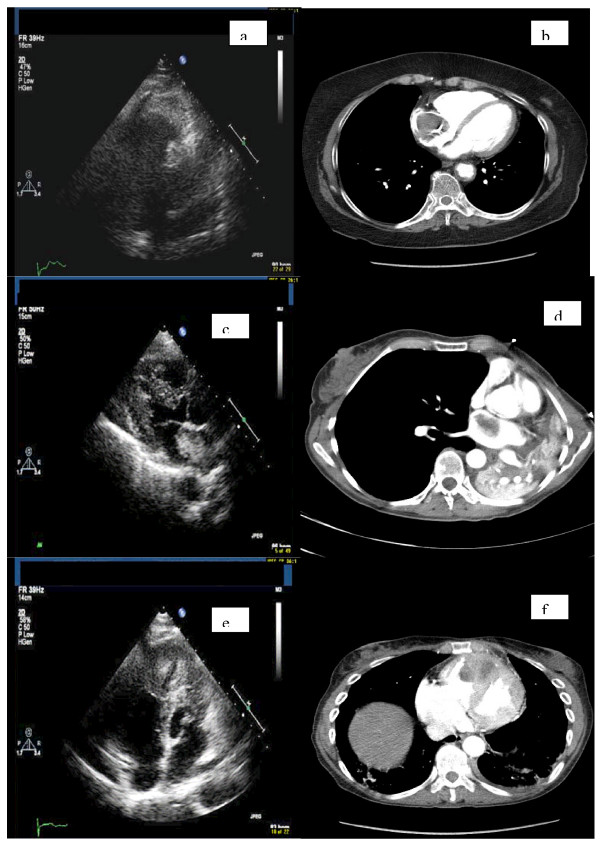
a. Echocardiographic image of case 1 showing the tumor in right atrium. b. CT image of case 1 showing the tumor. c. Echocardiographic image of case 2 showing the tumor in left atrium. d. CT image of case 1 showing the tumor. e. Echocardiographic image of case 3 showing the tumor in right ventricle. f. CT image of case 3 showing the tumor.
